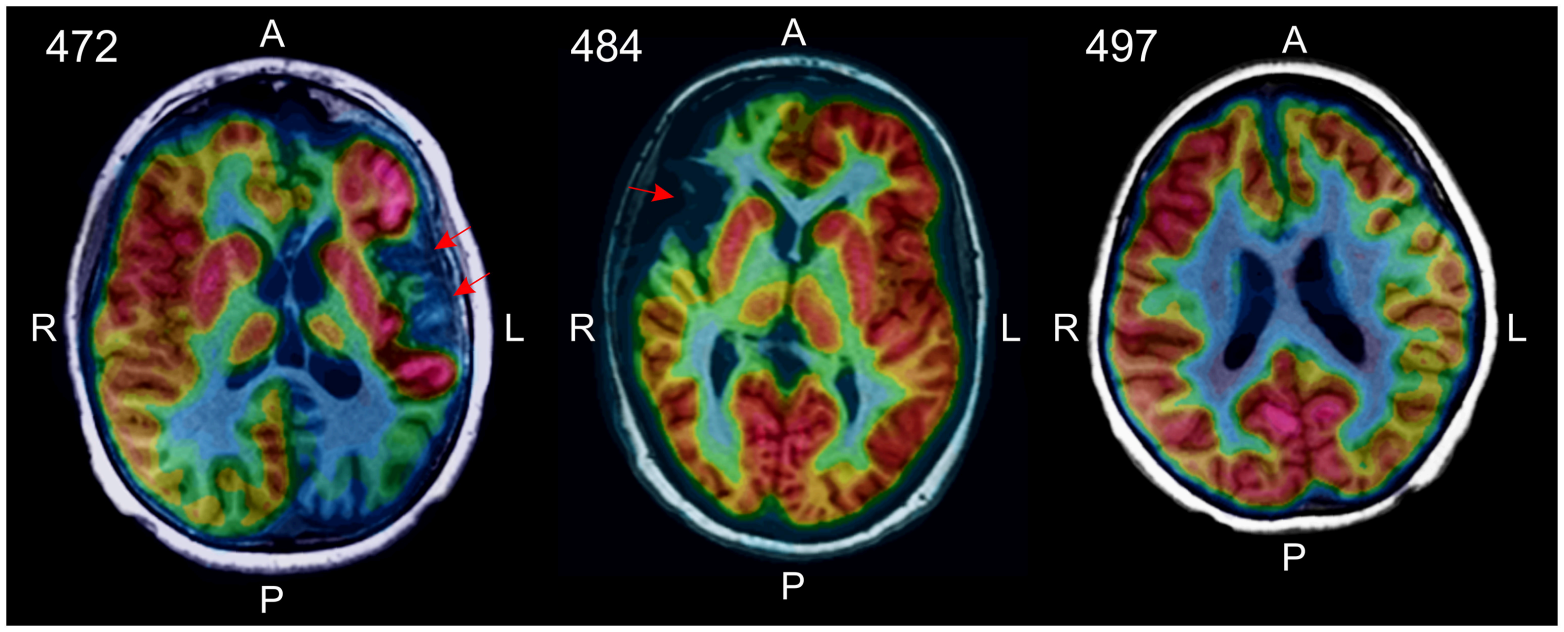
[^18^F]-deoxyglucose positron emission tomography (FDG-PET) identified areas of atrophy in RE cases 472 and 484 (red arrows). In RE case 497 there were no obvious signs of atrophy in the affected hemisphere, rather decreased glucose metabolism over the entire hemisphere was apparent.
